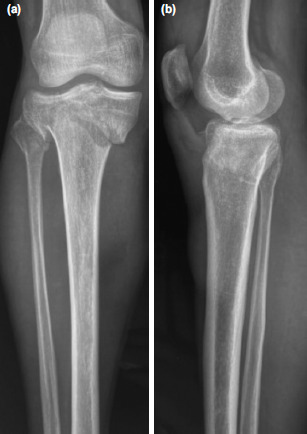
Case No.7, pre-operative radiographs of the knee showing Shatzker Type VI fracture. (a) Antero-posterior view. (b) Lateral view.
